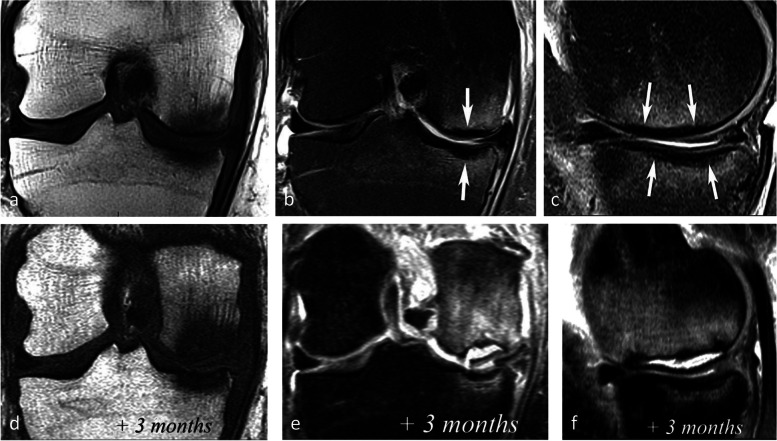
Negative prognostic value of low signal bone marrow on T2-weighted MR image. a The subchondral bone of the two medial femorotibial joint surfaces shows marked low signal intensity on a T1-weighted MR image. b, c Coronal and sagittal fat-suppressed T2-weighted MR images (b, c) show relatively wide and thick subchondral areas adjoining the articular surface, with low signal intensity (arrows), suggestive of SIF-ON. d–f Three months later, a significant bone marrow low signal intensity persists on T1-weighted image (d) and coronal and sagittal fat-suppressed T2-weighted images show pejorative evolution with a collapse of the tibial plateau and a wide subchondral bone dissection and chondral fracture in the lower pole of the condyle (e, f)
